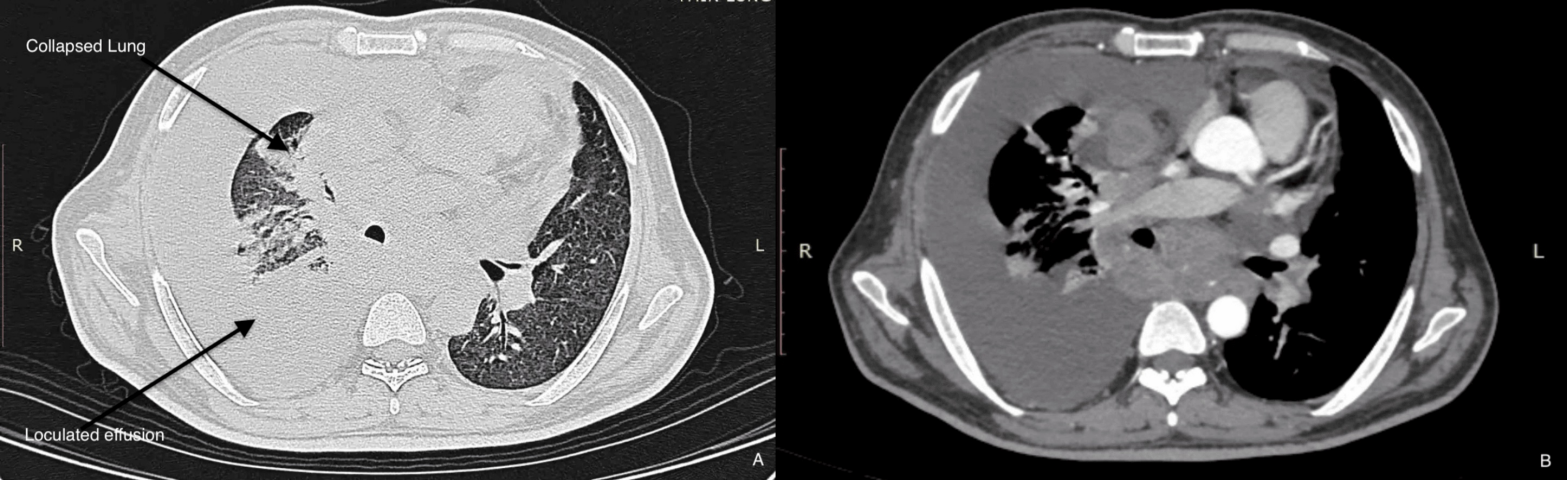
HRCT showing right moderate loculated pleural effusion with partial lung collapse HRCT: High-resolution computed tomography, A: Lung window, B: Mediastinal window
